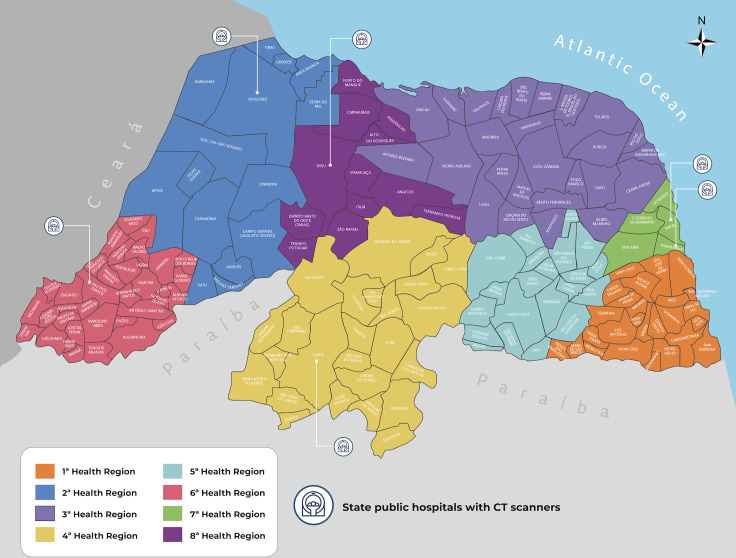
Distribution of Health Regions in Rio Grande do Norte. CT: computed tomography.
